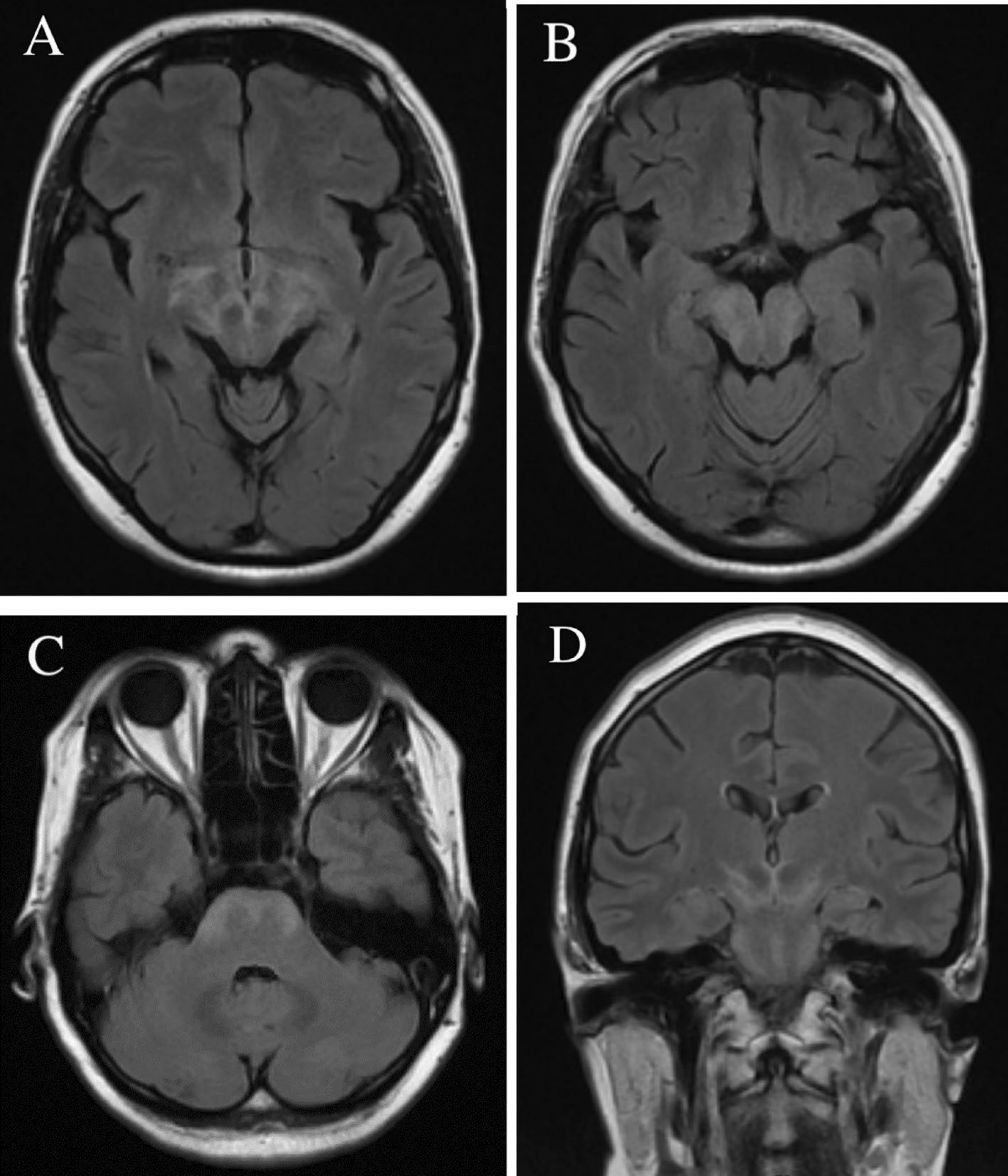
Head MRI of a patient diagnosed with Lyme neuroborreliosis (A–C axial T2 FLAIR, D coronal T2 FLAIR), showing confluent T2 hyperintense lesions involving basal ganglia, diencephalon, midbrain, and basal pons.
